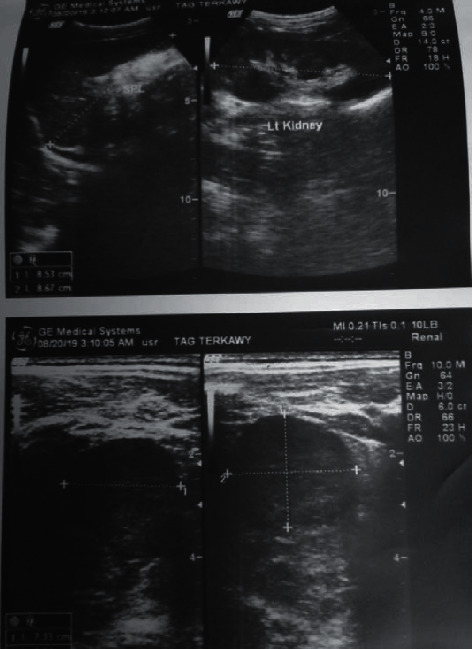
An abdominal ultrasound showed a well-defined rounded hypoechoic lesion with multiple tiny calcifications in the left suprarenal region measured 37 × 27 × 25 mm.
